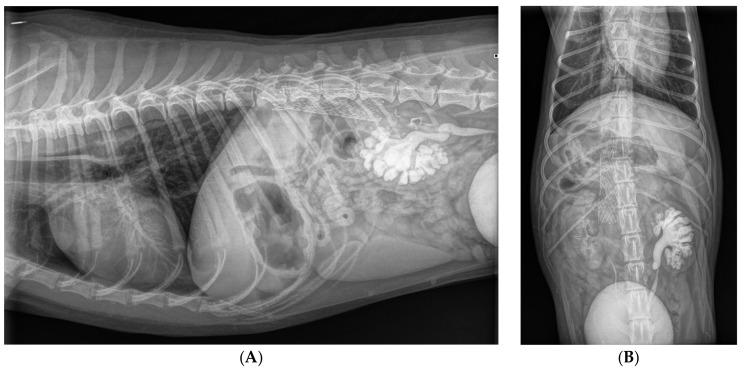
Radiographs in lateral (A) and in dorsoventral (B) projections made immediately after implantation of the stent in the right azygos vein. The stent is visible at the level of T12 to L1 vertebrae. As a result of renal excretion of the intravenously administered iodinated contrast material, the contrast is visible in the urinary bladder, both ureters and both kidneys. The left renal pelvis and the left ureter are moderately dilated as a result of an assumed compression of the retrocaval part of the left ureter.
